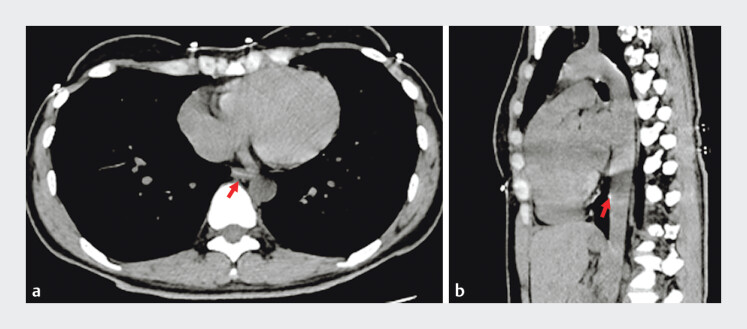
Computed tomography scan revealing the location of the fishbone (arrow).
